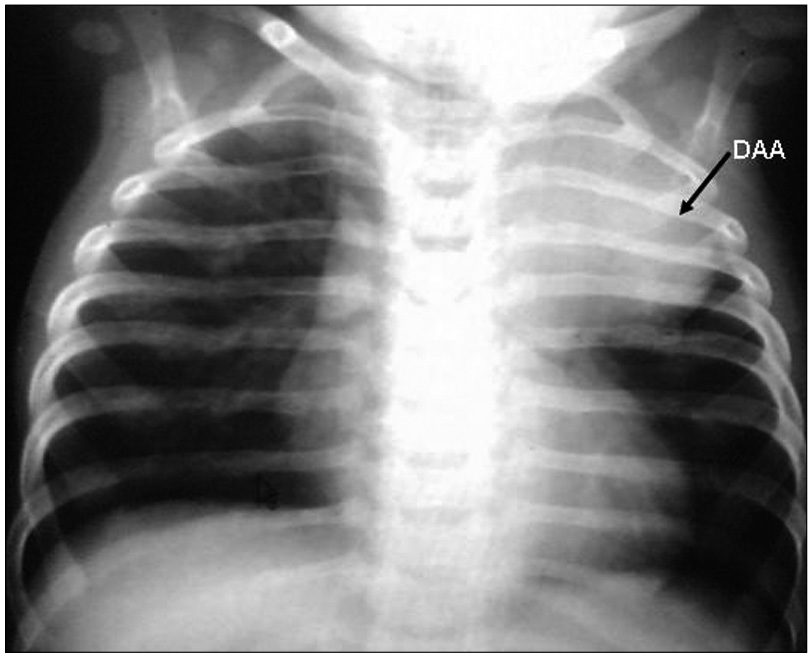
Chest X-ray (PA view) showing homogenous rounded opacity in the left upper thorax produced by the ductal aneurysm, normal lung parenchyma, normal pulmonary blood flow, and there was no cardiomegaly. DAA-ductus arteriosus aneurysm
